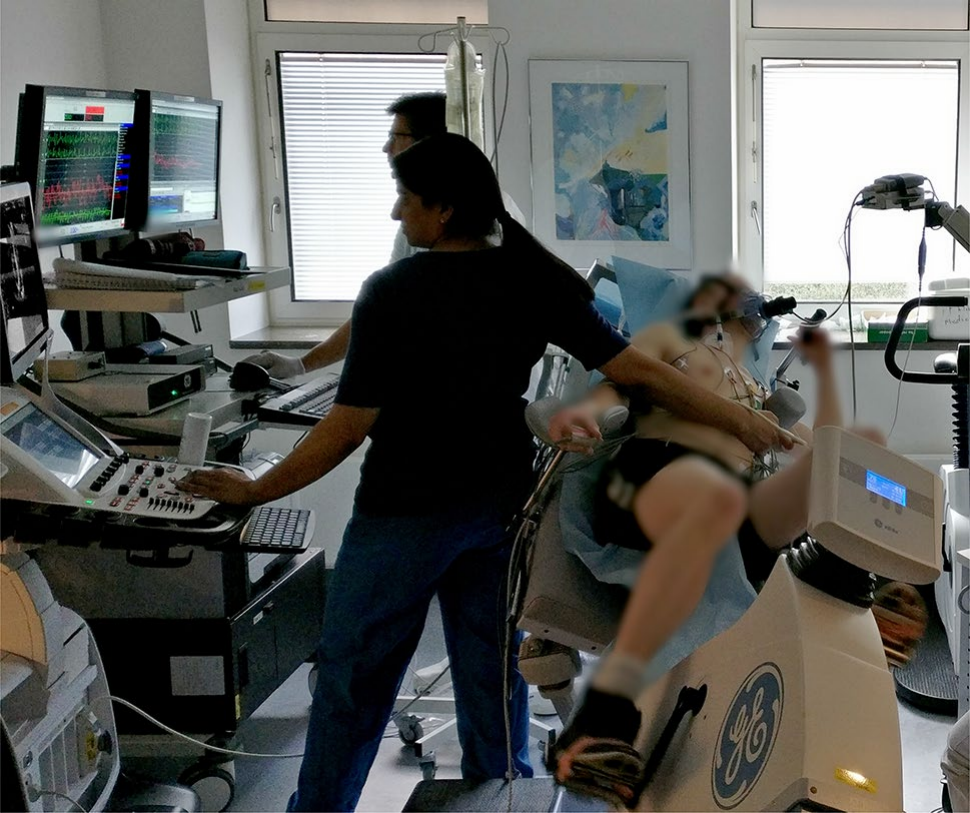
Examination setup. An image of the examination setup. The participant is installed on a semi-supine ergometer wearing a mask to measure direct oxygen consumption. Heart rate, saturation and blood pressure are constantly monitored. The cardiopulmonary exercise test is simultaneously performed with a stress echocardiographic examination and invasive hemodynamic measurements through an already placed Swan Ganz catheter. The participant exercises until maximal exhaustion with work load increments every three minutes. Stress echocardiographic images and pressure measurements were obtained during the last 1.5 min of each 3-min load increment
